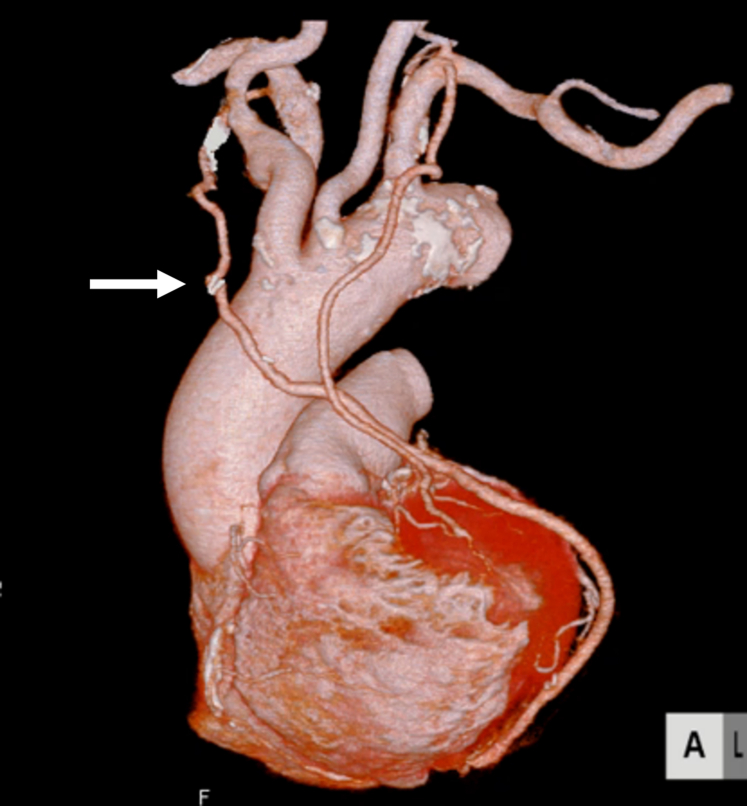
Postoperative computed tomography revealed all graft patency. The *arrow* indicates the anastomotic site between the right internal thoracic artery and the great saphenous vein.
